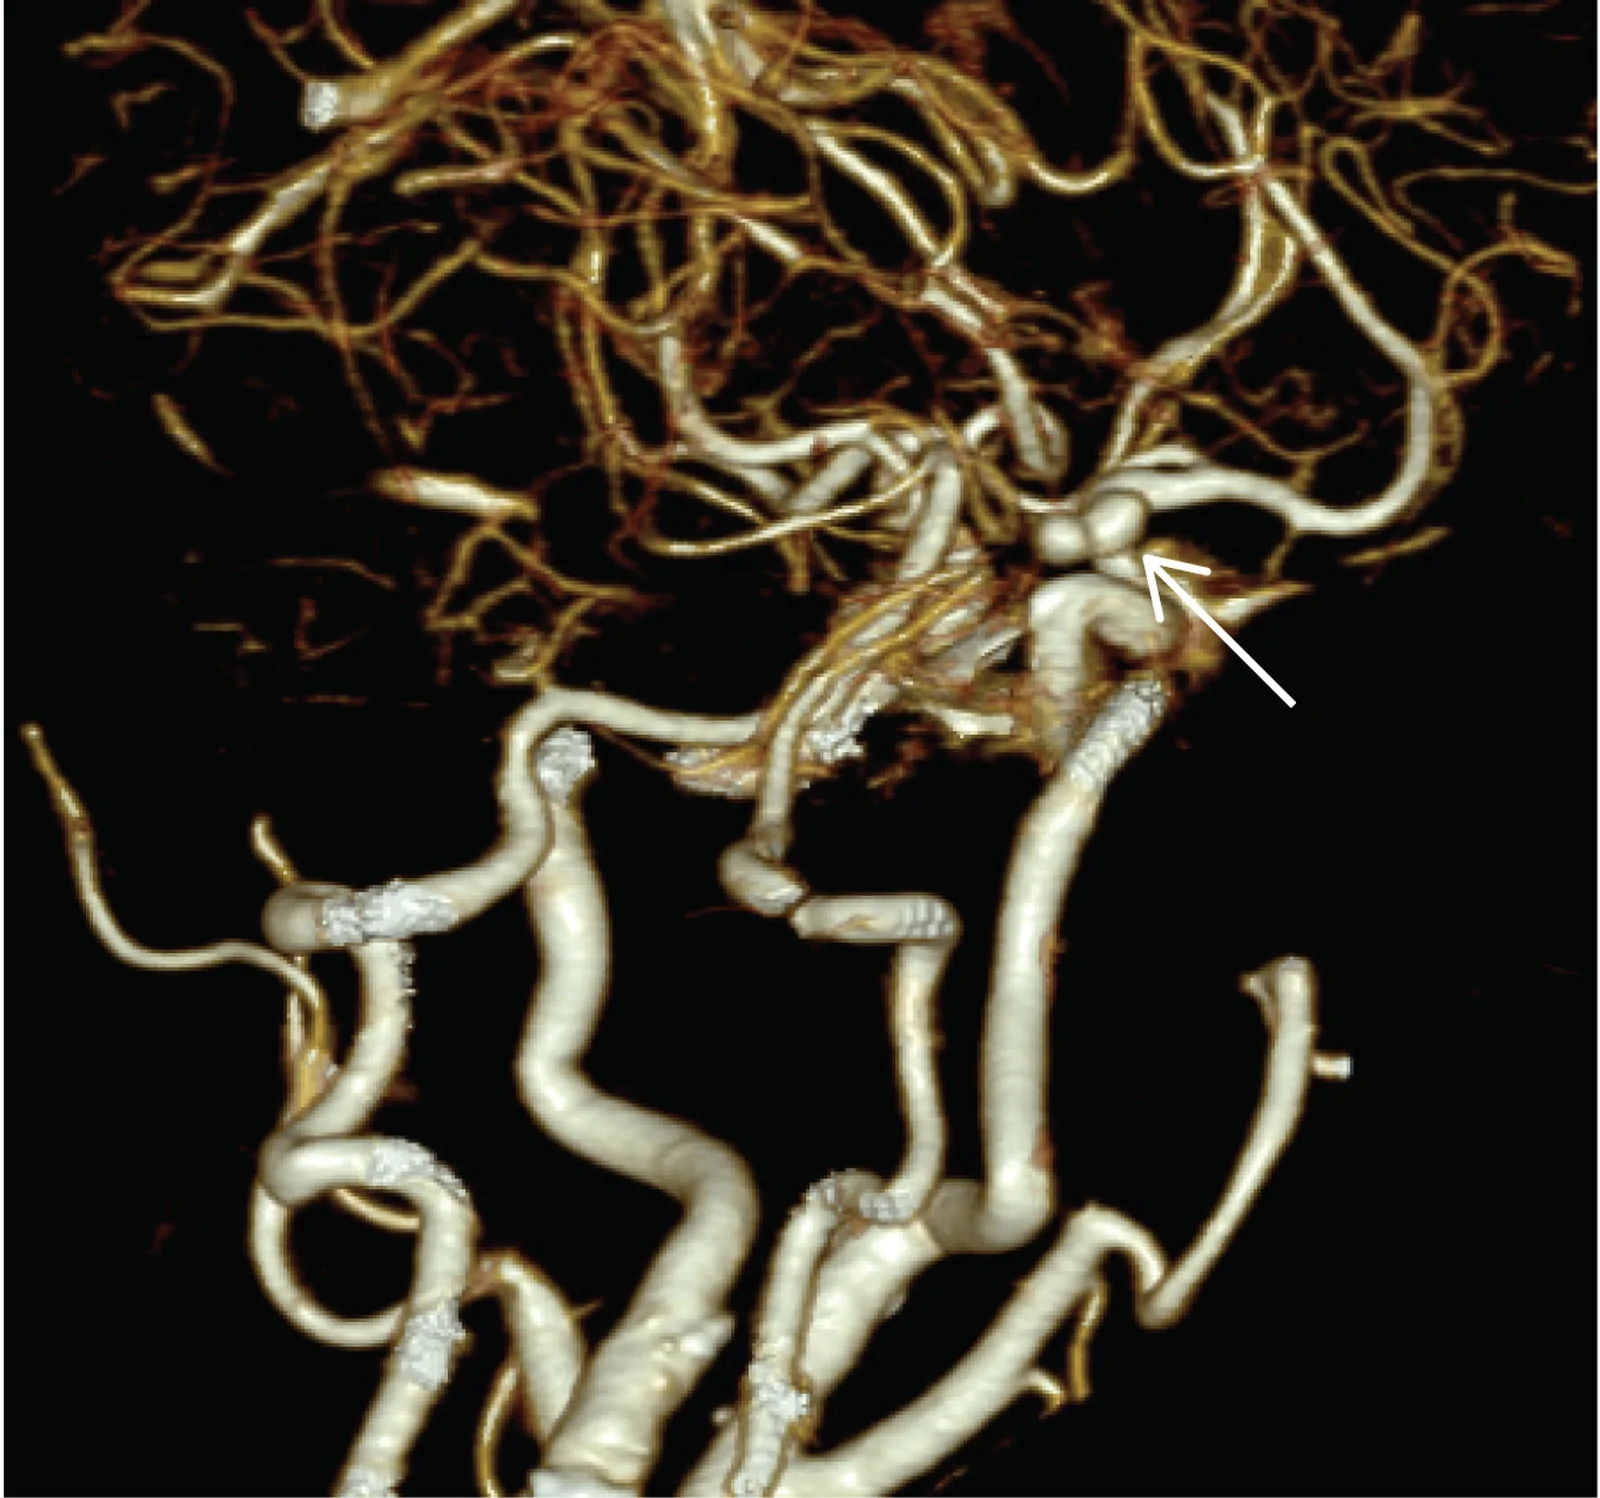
Three-dimensional reconstruction of CT angiography showing an aneurysm at the junction of the posterior communicating artery and the internal carotid artery.
